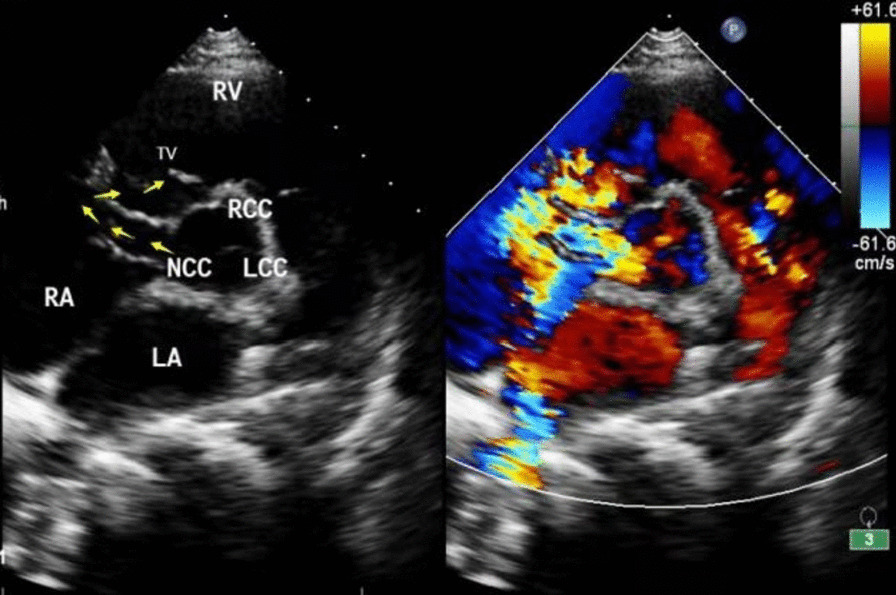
Echocardiography with colour Doppler showing a high-velocity multicoloured (aliasing) mosaic of blood flow from the NCC to RA. The yellow arrow represents the shunt. NCS: noncoronary sinus; RCS: right coronary sinus; LCS: left coronary sinus; RA: right atrium; RV, right ventricle; LA: left atrium; TV, tricuspid valve
